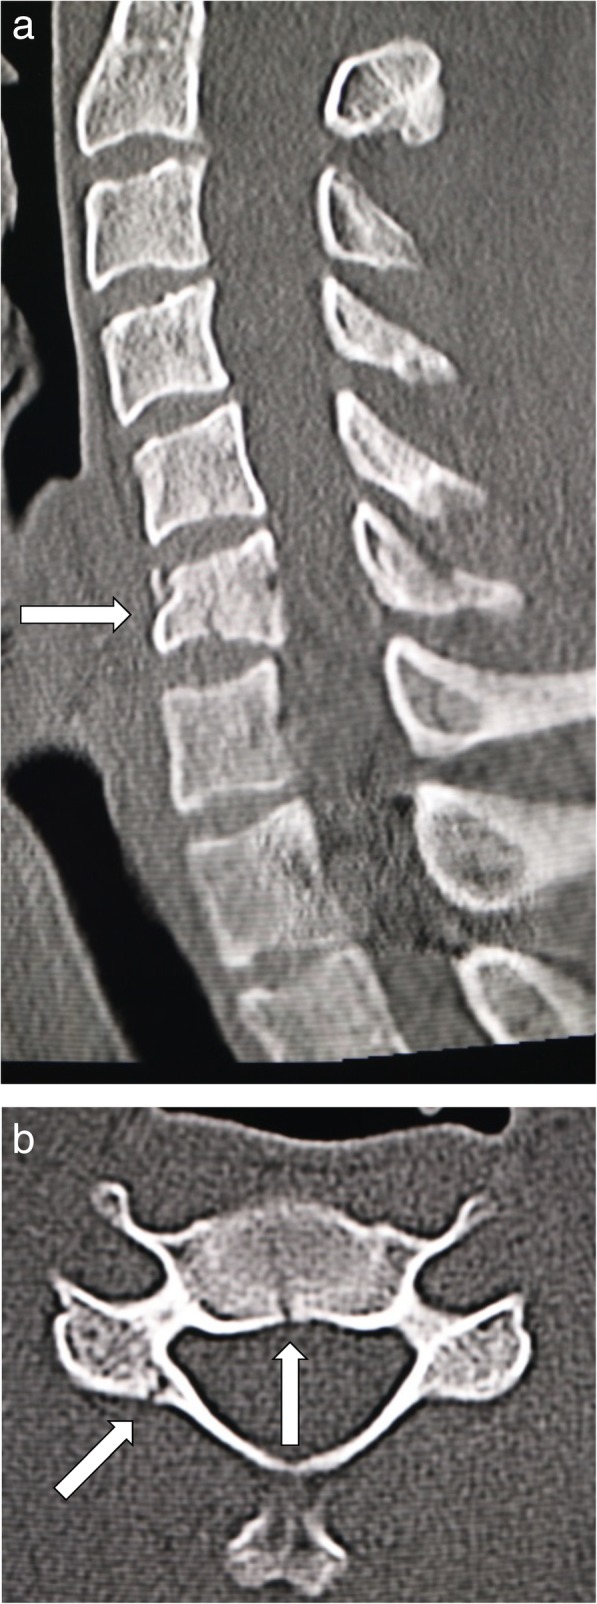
A radiographic example of a patient included in this study. a The patient suffered from compressive-flexion vertebral fracture with preserved cervical alignment. b The vertical fracture of the vertebra and the right laminar fracture occurred concurrently in this patient
